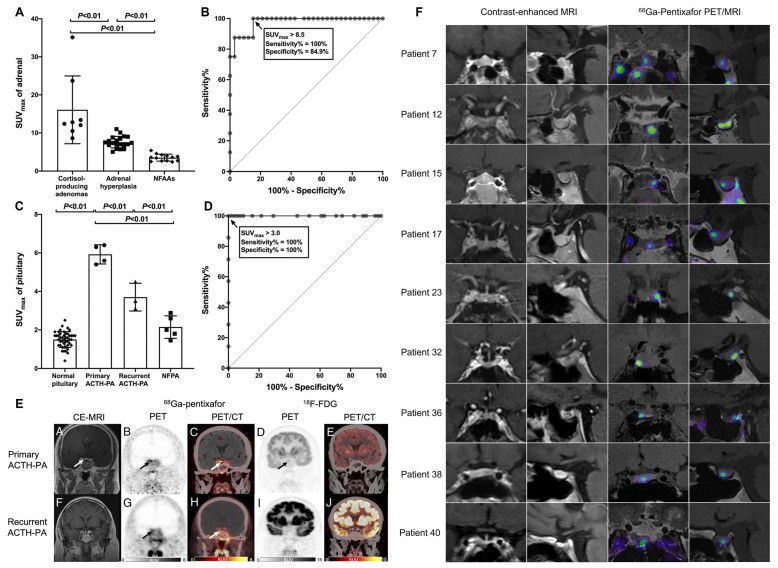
Clinical application of [^68^Ga]Pentixafor in CS. (A) SUVmax comparison of [^68^Ga]Pentixafor in cortisol-producing adenoma, adrenal hyperplasia, and nonfunctioning adrenal adenoma. (B) ROC analysis of [^68^Ga]Pentixafor SUVmax for discriminating cortisol-producing adenoma from adrenal hyperplasia and nonfunctioning adrenal adenoma. (C) SUVmax distribution of [^68^Ga]Pentixafor across normal pituitary, primary/recurrent ACTH-producing pituitary adenoma, and nonfunctioning pituitary adenoma. (D) ROC evaluation of [^68^Ga]Pentixafor SUVmax for differentiating ACTH-producing pituitary adenoma from nonfunctioning pituitary adenoma and normal pituitary. (E) A 41-year-old man with Cushing's disease, pathologically confirmed as a right-sided ACTH-producing pituitary adenoma (5 mm maximum diameter). Contrast-enhanced MRI demonstrated a hypointense microadenoma in the right pituitary wing (arrow). [^68^Ga]Pentixafor PET/CT coronal PET and fusion images revealed focal uptake (SUVmax 6.3; arrows), while [^18^F]FDG PET/CT showed only mild diffuse pituitary uptake without distinct foci (arrows). A 41-year-old woman with recurrent right-sided ACTH-producing pituitary adenoma (3 mm maximum diameter). Contrast-enhanced MRI was negative. [^68^Ga]Pentixafor coronal PET and fusion images demonstrated increased uptake (SUVmax 4.5; arrows), whereas [^18^F]FDG coronal PET and fusion images showed no abnormal uptake in the pituitary fossa. Adapted with permission from 81, copyright © 2022, Wolters Kluwer. (F) Coronal and sagittal contrast-enhanced MRI and corresponding [^68^Ga]Pentixafor PET/MRI scans in study participants show examples of negative cases (no detected lesion). Adapted with permission from 105, copyright © 2024, Radiological Society of North America.
